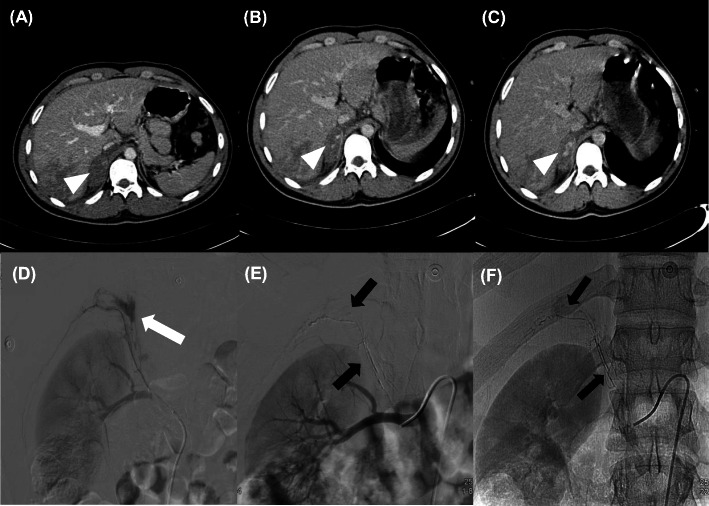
(a, b, c) CT image venous phase (a) and arterial phase (b, c) after endovenous contrast injection, depicting contrast deposition inside the right adrenal gland (white arrowhead). (d) DSA with injection right renal artery showing contrast extravasation (white arrow). (e) DSA with injection right adrenal artery showing glue cast (black arrow) in a subtracted image in the adrenal territory after embolization with complete superseletiva exclusion of the injured vessel from the circulation. (f) Subtraction image showing glue cast (black arrow)
